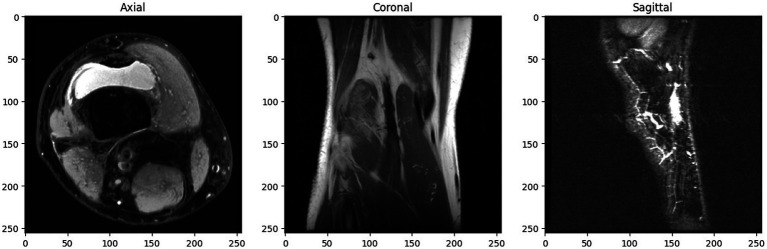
Multi-view knee MRI slice of MRNet dataset.
